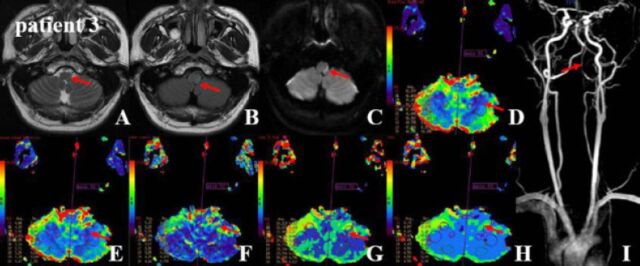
MRI-T2WI, Flair and DWI demonstrated a-c) high signal intensities in the left lateral medulla. d-h) Dynamic susceptibility contrast-enhanced-perfusion weighted imaging demonstrated left lesion ROI low CBF, low CBV, delayed MTT, and deceased Tmax on the VAH side. The representative rigid quantitative thresholds in the ROIs of 11 and 12 are shown in Table 2. i) Constrast-enhanced magnetic resonance artery indicated left VAH and minor stricture in the V4 segment of the left vertebral artery. VAH - vertebral artery hypoplasia, CBF - cerebral blood flow, CBV - cerebral blood volume, TTP - time to peak, MTT - mean transit time, ROI - region of interest
